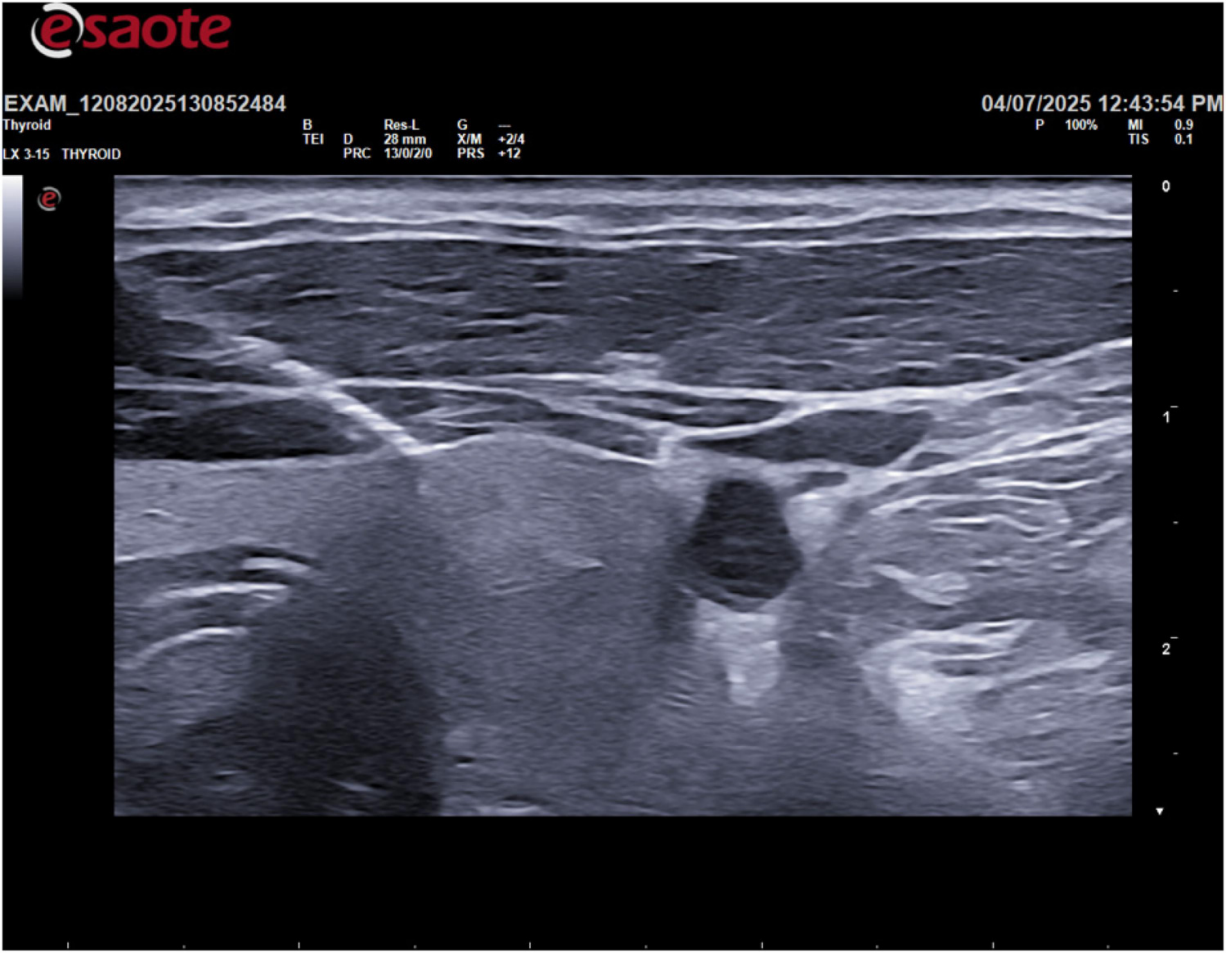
Ultrasound-guided fine-needle aspiration (FNA).
